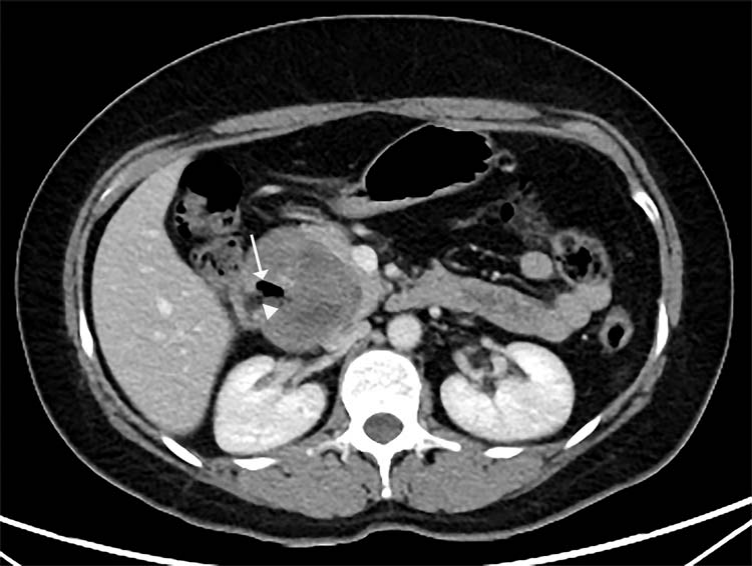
CT scan showing that tumour reduced to 6 cm, containing an air cavity (white arrow) and communicating with the duodenal lumen (arrowhead).
